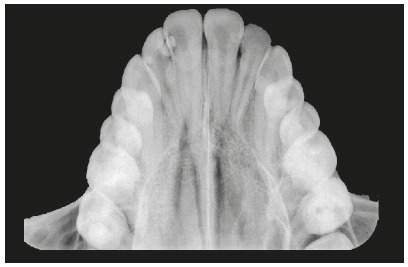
Initial occlusal radiograph.
